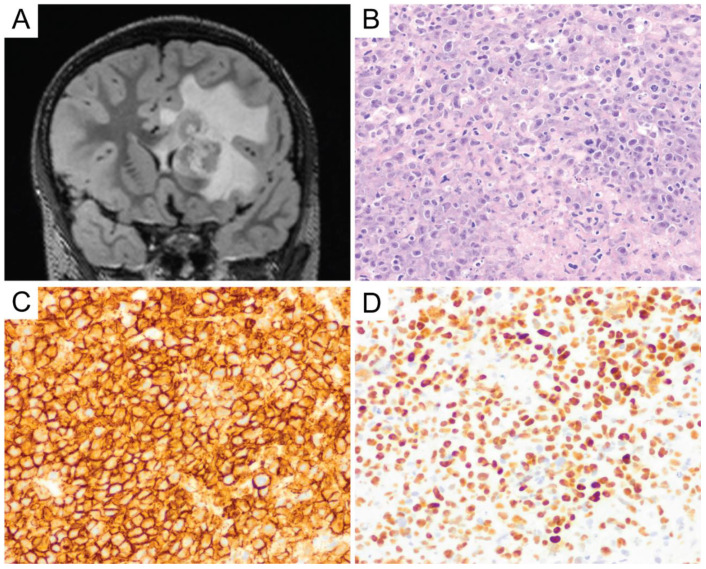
Pediatric primary central nervous system lymphoma (PCNSL). Pediatric PCNSL typically presents as a contrast-enhancing and T2 hyperintense lesion with associated parenchymal edema on MRI (A). The tumor cells are large with irregular nuclei and scant cytoplasm, often with associated necrosis (B, original magnification ×400). The tumor cells are positive for CD20 (C, original magnification ×400). The lymphoma cells in this example were of germinal center phenotype, showing no expression of CD10 (not shown) and MUM1 (not shown), and were positive for Bcl-6 (D, original magnification ×400).
